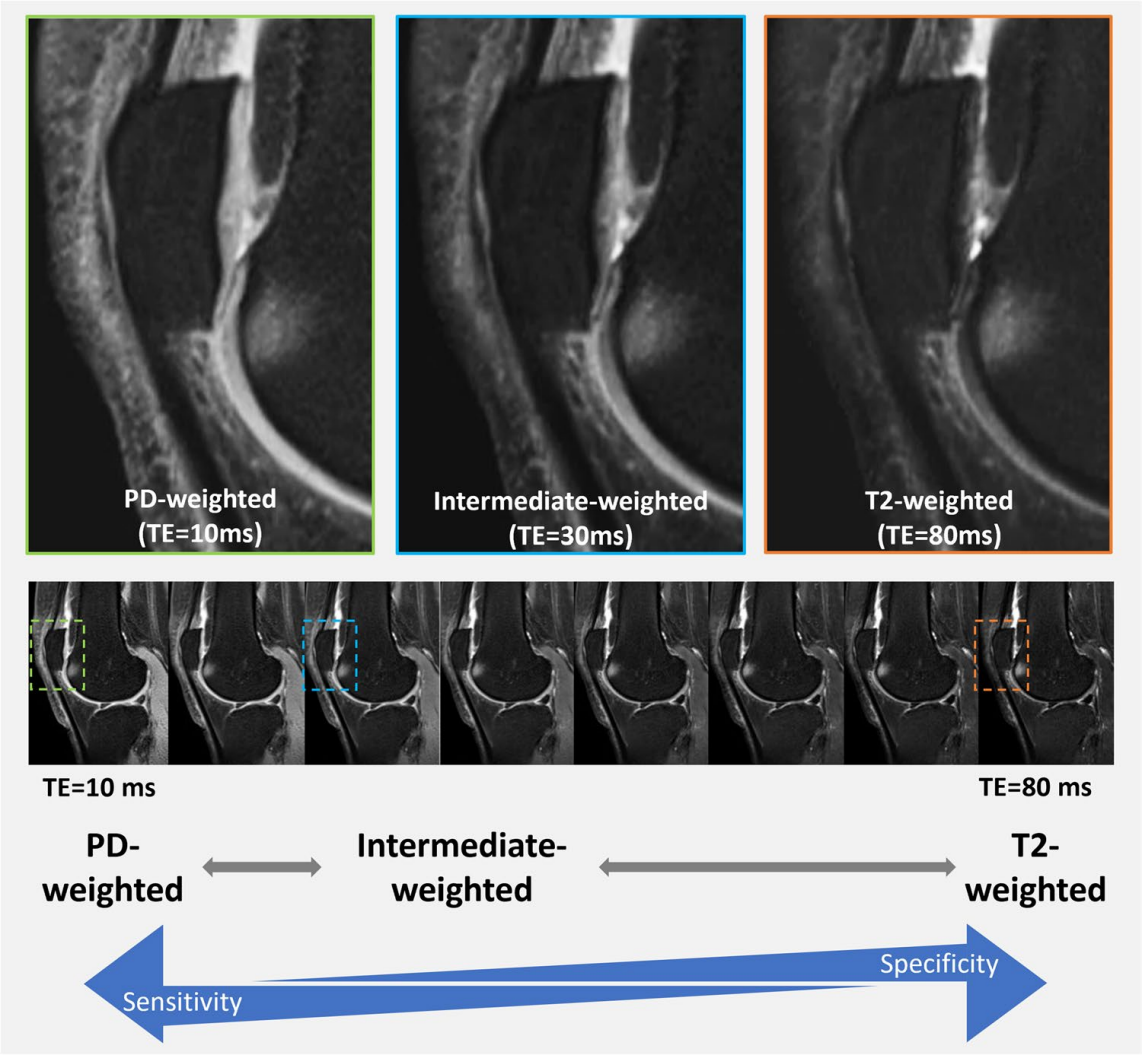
Sagittal fat-suppressed MR images with serial TEs ranging from 10 to 80ms (images from lower row) synthesized from a single multi-TE T2 map acquisition (GRAPPATINI ®) obtained on a 3T MRI unit, with selected zoomed in images (upper row) corresponding to PD-weighted, intermediate-weighted and T2-weighted. Images are windowed identically. Note that with increasing TE values, signal of lower T2 tissues, including cartilage, decays, but the contrast between fluid and cartilage increases. The higher the T2-weighting (i.e., the higher the TE), the lower the sensitivity, but the higher the specificity to fluid signal
